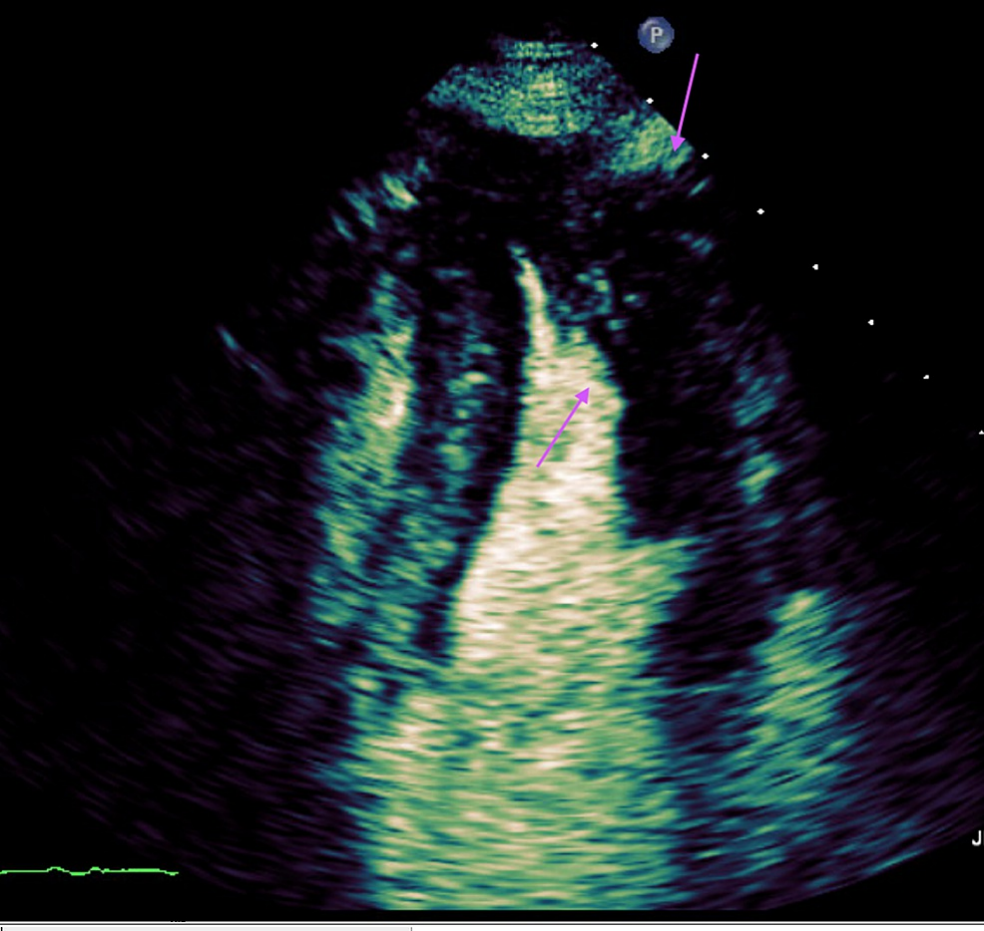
Transesophageal echocardiogram (TEE) with contrast demonstrating apical hypertrophy and end-diastolic obliteration with an ejection fraction (EF) of 65%-70%
